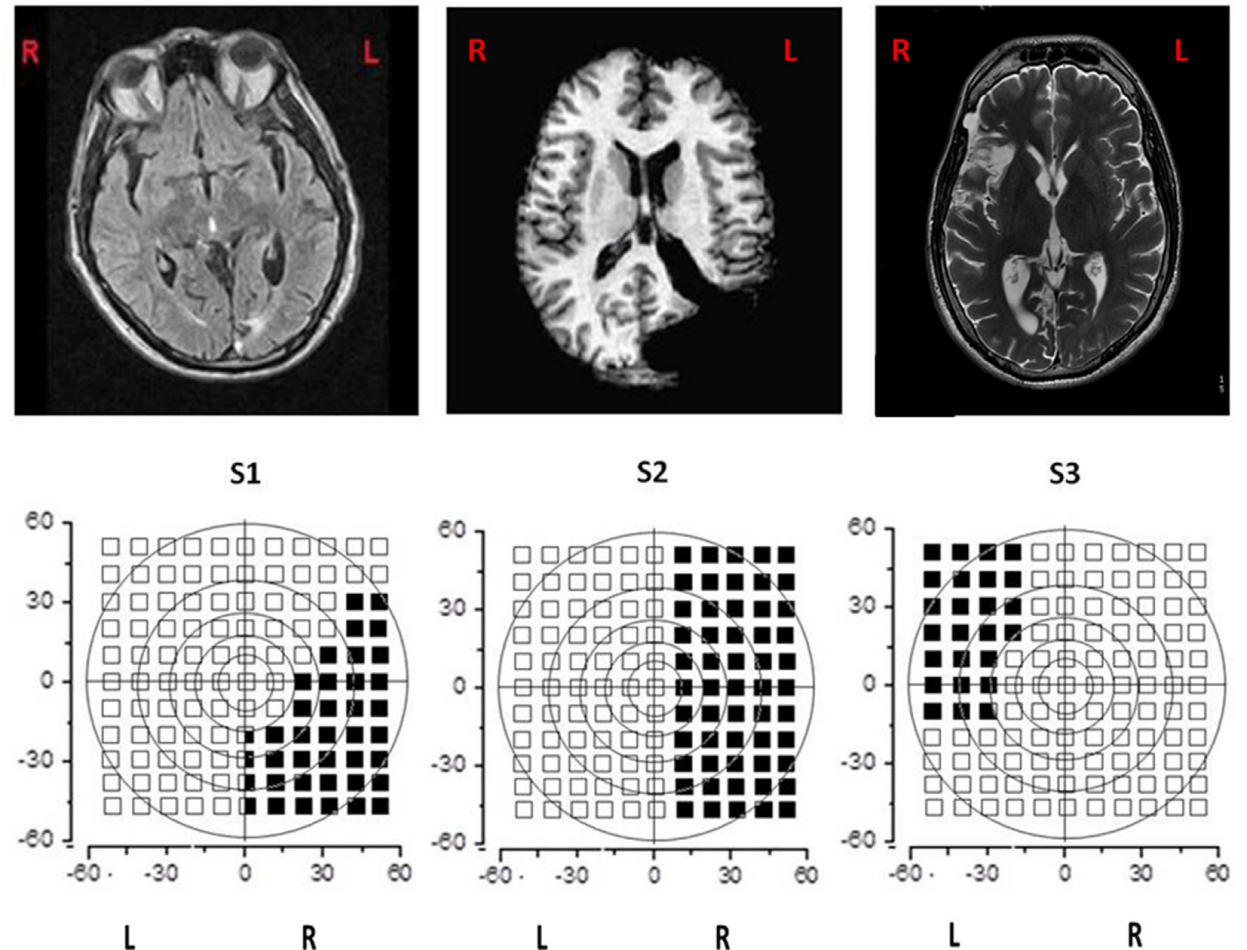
Magnetic resonance imaging of the brain and visual field campimetry of S1, S2, and S3.
